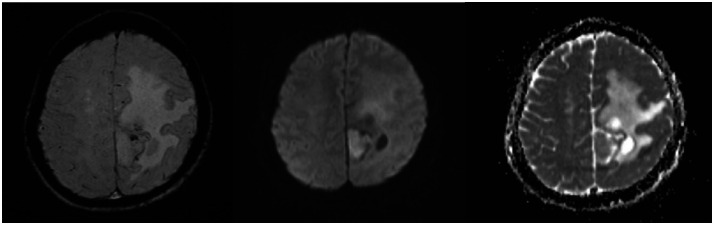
MRI of an atypical meningioma demonstrating areas of susceptibility on SWI (left), diffusion restriction on DWI (center), and corresponding low signal on the ADC map (right).
